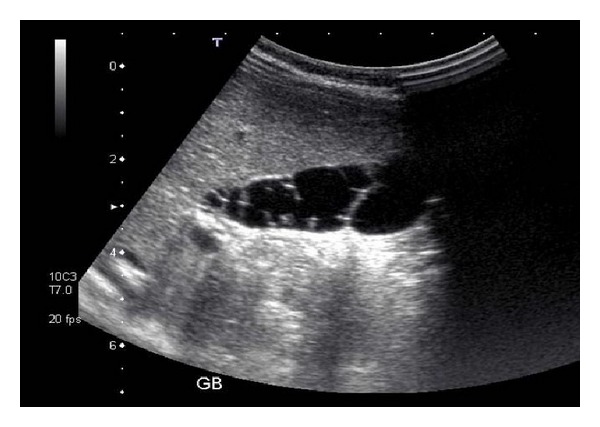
Ultrasound image demonstrating multiple fine septations within the gallbladder.
